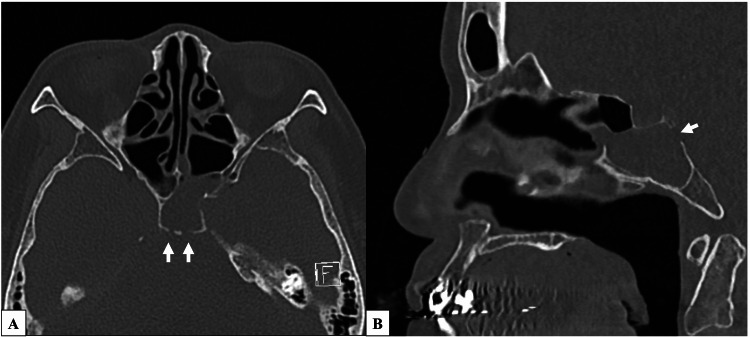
Preoperative computed tomography (CT) images of the area around the clivus. Computed tomography (CT) scan of a 0.5-mm slice revealed multiple bony defects in the upper clivus. White arrows indicate the bony defects.
